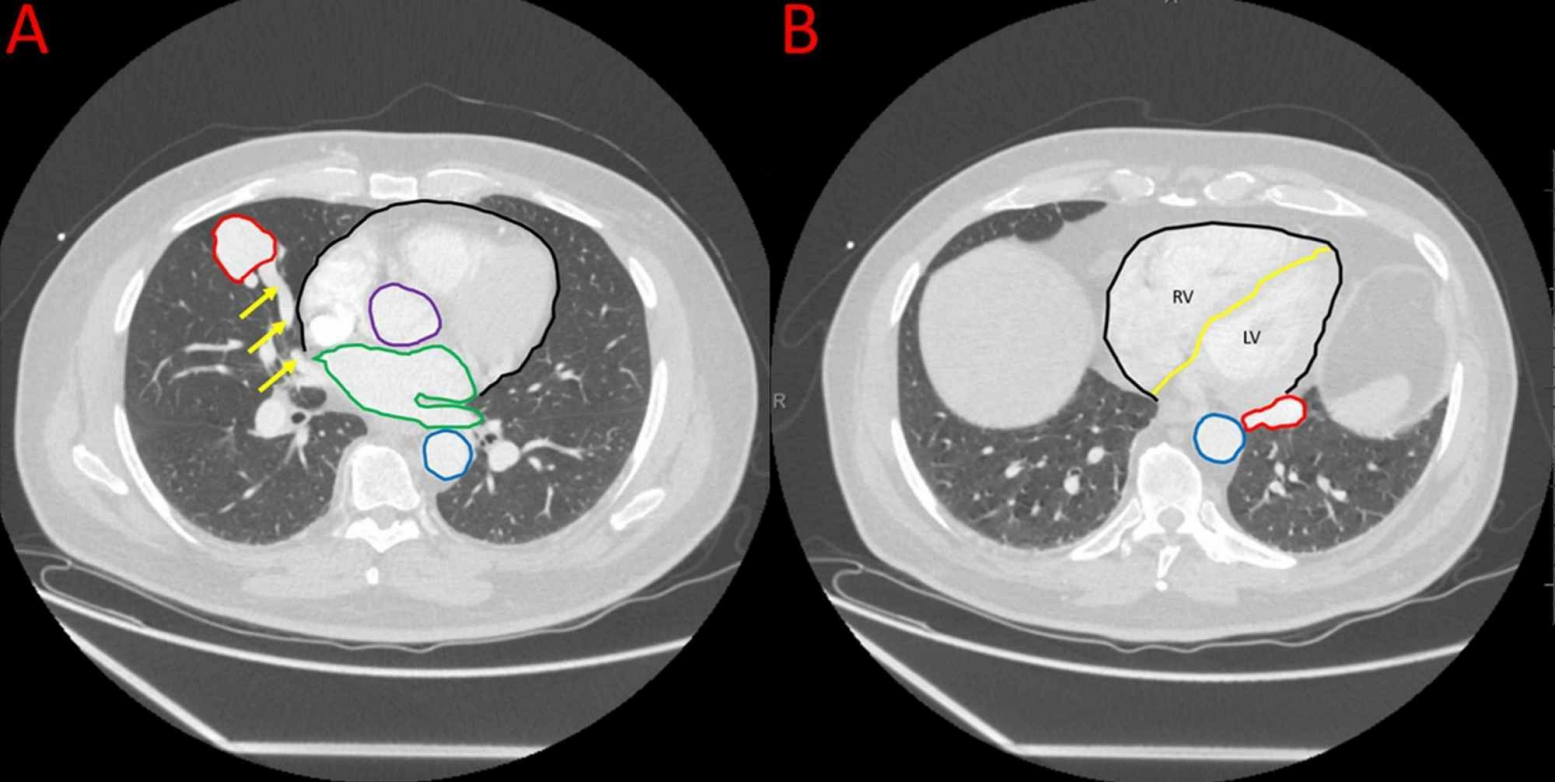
CT of the Chest Showing Two Pulmonary Artery AVMs in the Axial Field of View CT of the chest with contrast in the axial view at different levels, reveals two pulmonary arteries to pulmonary vein fistulas, or AVMs (red circles) with one in the right middle lobe (A) and one in the left lower lobe (B). The aneurysmal component in the right middle lobe (A) measures 2.6 cm × 2.5 cm × 2.8 cm. The AVM is supplied by a single segmental branch of the right pulmonary artery (yellow arrows). The pulmonary veins draining into the left atrium are in the axial field of view, demonstrated by the green outline. The cardiac border can be seen in the axial field, demonstrated by the black outline. The ascending and descending aorta can be seen in the axial field of view, demonstrated by the purple and blue outlines, respectively. The second AVM (B) originates from a single-segmental branch of the pulmonary artery in the left lower lobe and contains a multilobulated aneurysm measuring approximately 1.6 cm × 3.0 cm × 1.7 cm. The cardiac border can be seen in the axial field of view, demonstrated by the black outline. The right and left ventricles of the heart can be seen in the axial field of view, demonstrated by the letters “RV,” and “LV,” respectively. The descending aorta can be seen in the axial field of view, demonstrated by the blue outline. AVM: arteriovenous malformation.
